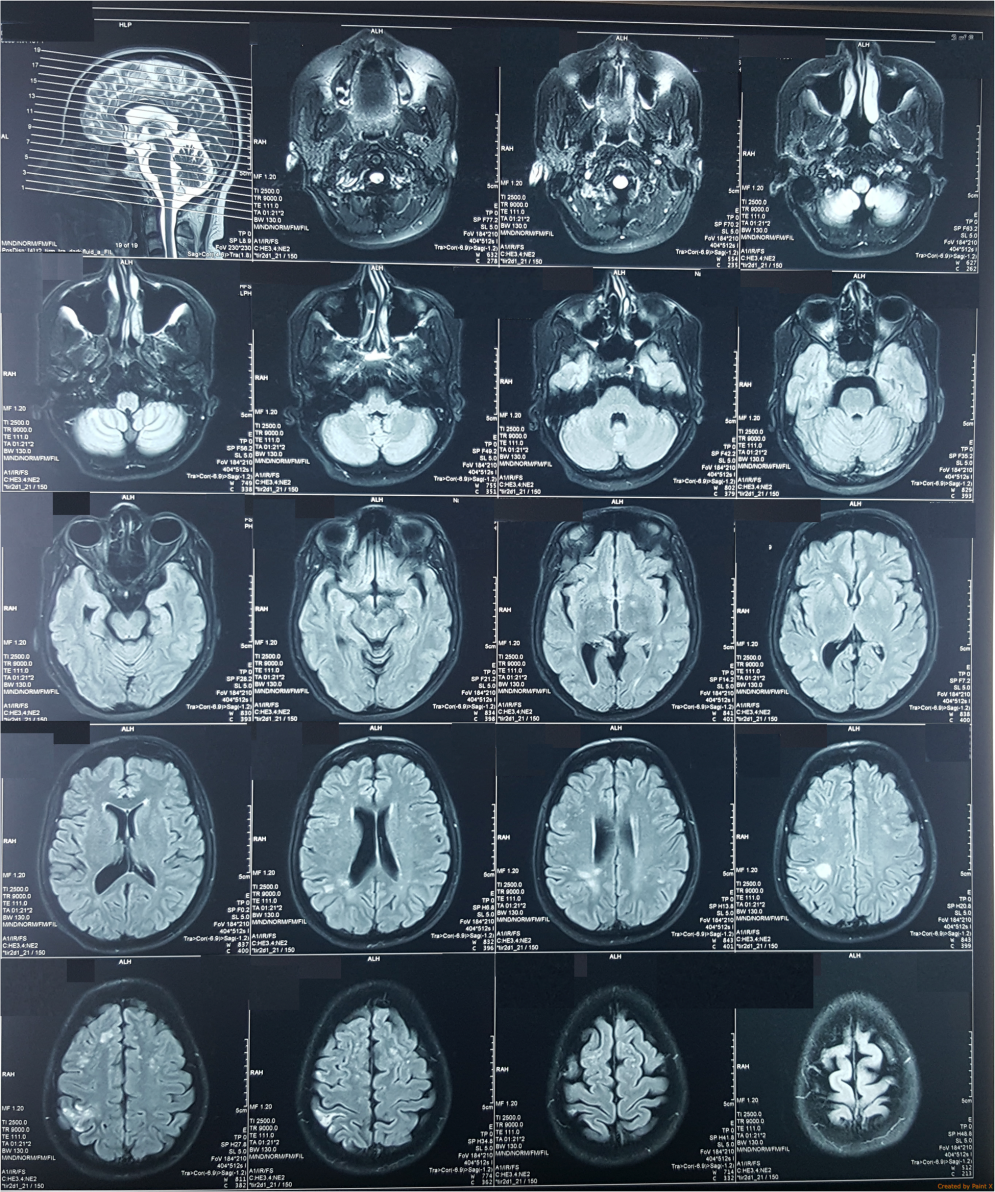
FLAIR images showing multiple, focal hyper intensities with partially restricted diffusion, suggestive of acute infarcts in the right parietal lobe
